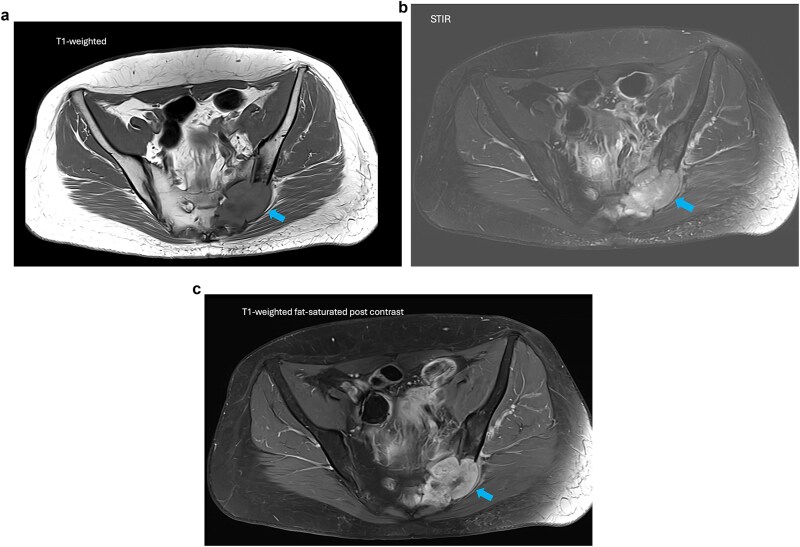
MRI of the pelvis. (a) Axial T1-weighted image demonstrating a lobulated low-signal mass centered in the left sacrum. (b) Axial STIR sequence showing heterogeneous high signal intensity with osseous destruction and soft tissue extension. (c) Axial T1-weighted fat-saturated post-contrast image demonstrating heterogeneous enhancement with small internal foci of necrosis.
